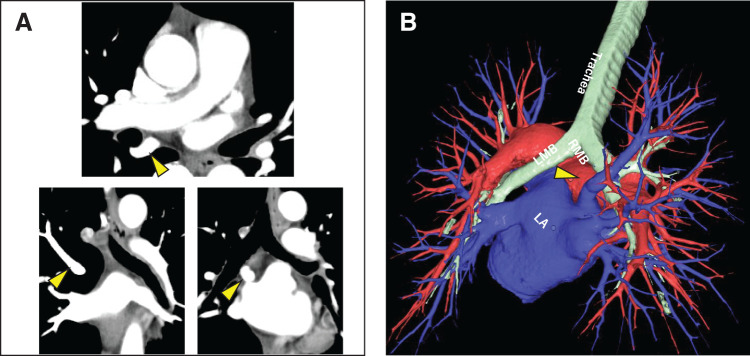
(A, B) Preoperative contrast-enhanced computed tomography and 3-dimensional (3D) imaging using Synapse Vincent (Fuji Photo Film Co., Ltd. ) showing the right top pulmonary vein behind the right main bronchus (yellow arrowhead). LMB, left main bronchus; RMB, right main bronchus; LA, left atrium
